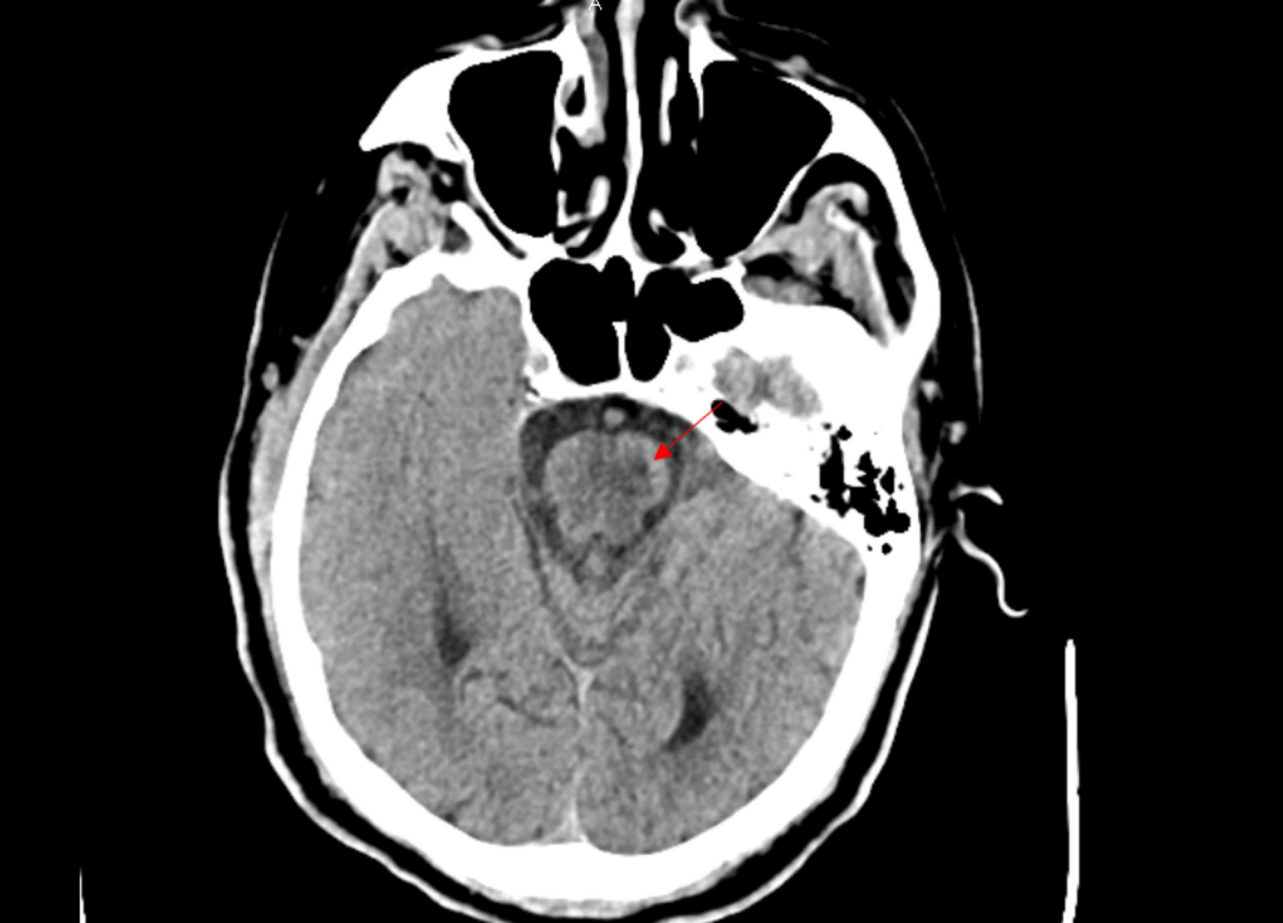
Case 3 head CT on April 25, 2020
